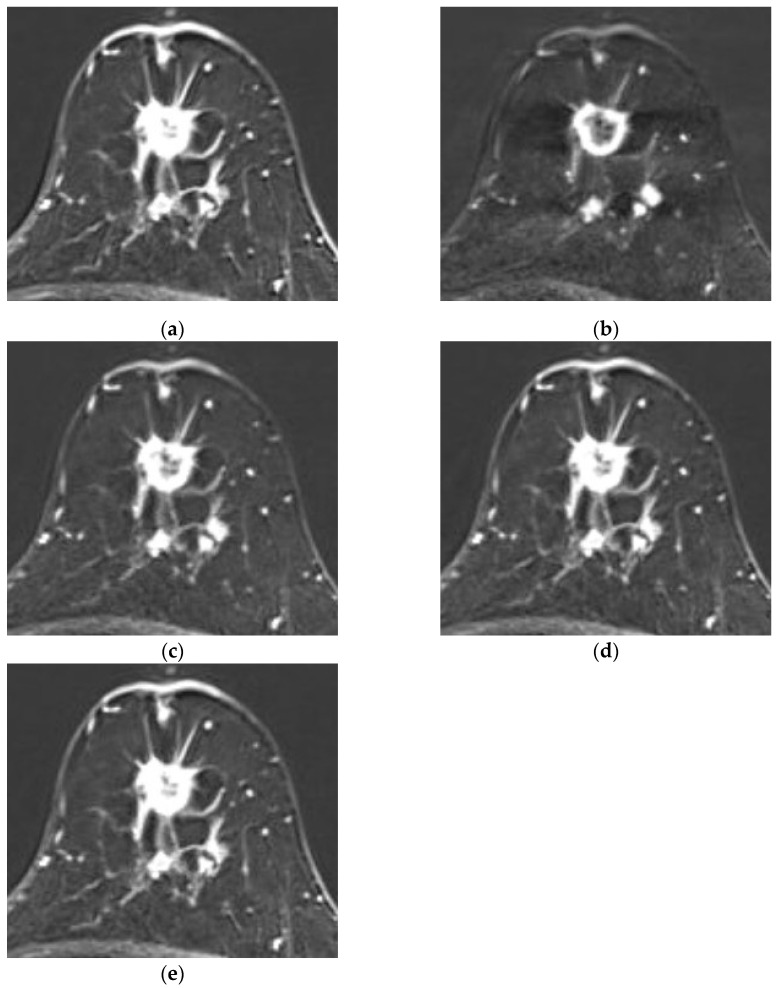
Representative case of HER2-low breast cancer with recurrence. Both the MRI-alone and combined models correctly predicted recurrence. A 50-year-old woman with heterogeneously dense fibroglandular tissue and moderate background parenchymal enhancement. Axial contrast-enhanced T1-weighted MR images at (a) 1 min, (b) 2 min, (c) 3 min, (d) 4 min, and (e) 5 min post-contrast administration show a 1.8 × 1.6 cm irregular-shaped, spiculated-margin, rim-enhancing mass located at the 12 o’clock position of the left breast. An additional 8 mm enhancing mass is seen posterior to the main lesion, with two other 8 mm enhancing masses laterally that appear interconnected. Although not shown in the images, another enhancing lesion was present in the upper inner quadrant (UIQ), confirming multicentric disease. Both models successfully predicted this recurrence case.
